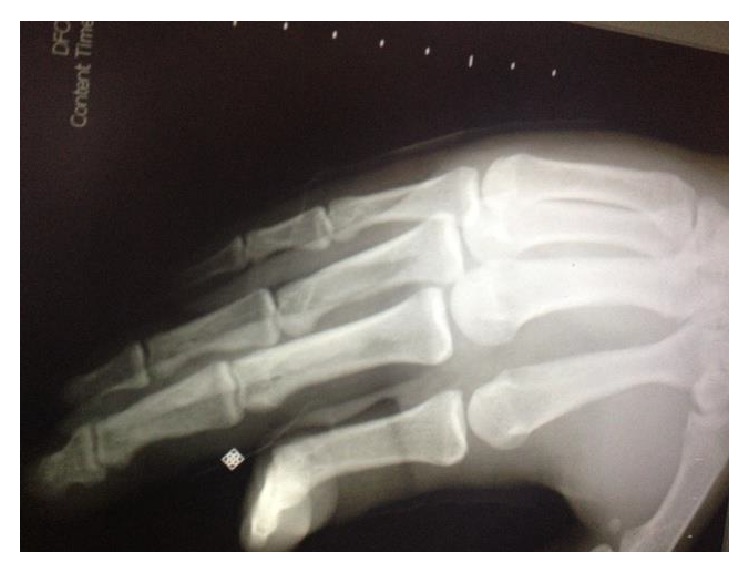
X-ray showing reduced finger.
